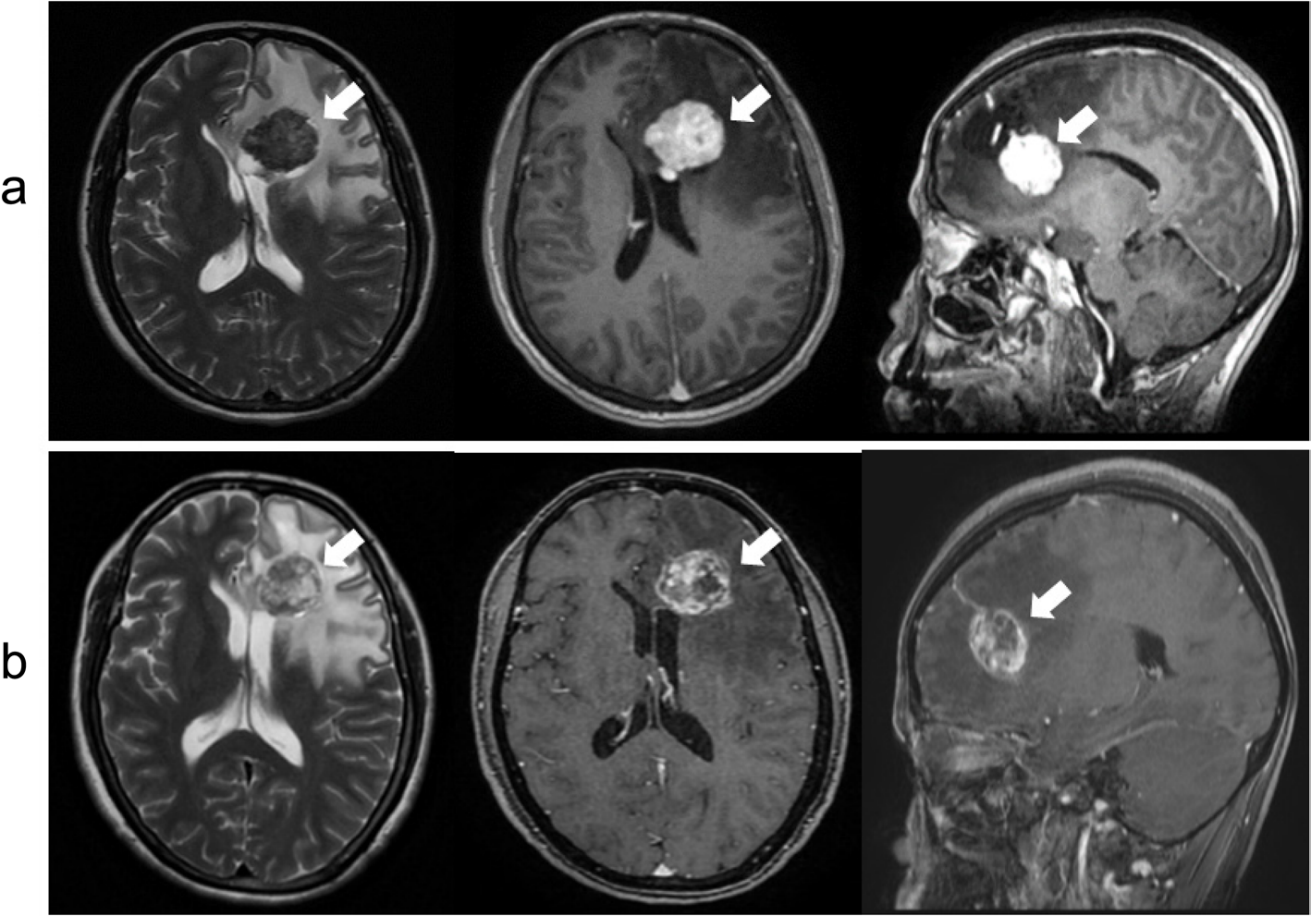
Pre and post radiosurgery Cerebral metastatic lesion (a): Pre-radiosurgery MRI showing the left frontal lesion with marked contrast enhancement (upper row). b: Three months post-treatment MRI showing no significant change in tumor dimensions but a reduction in contrast-enhanced portion and an increase in the necrotic part (lower row)
